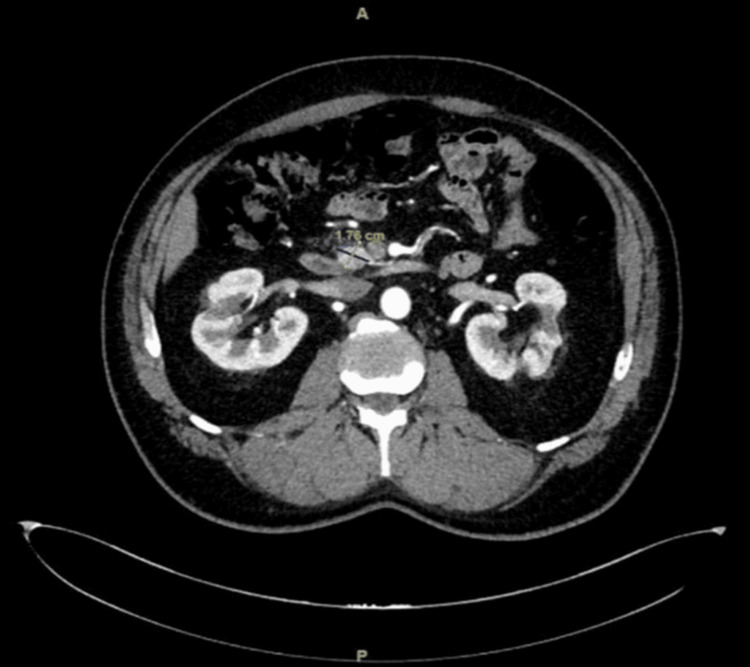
Three-year follow-up CT scan CT scan from February 2022, three years post-SBRT, demonstrating further reduction in tumor size to 2.2 × 1.8 cm. CT: Computed tomography; SBRT: Stereotactic body radiation therapy
